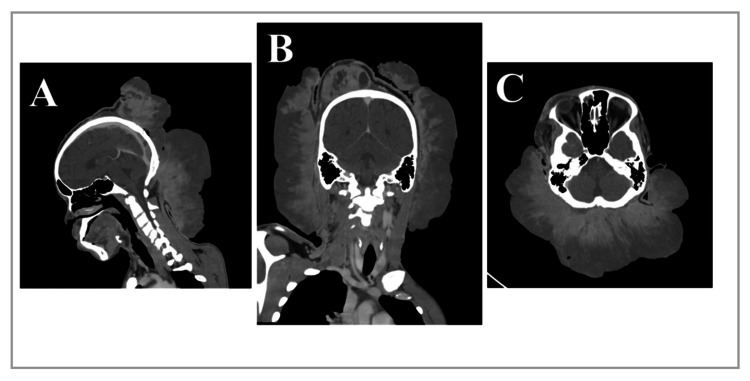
Cranial CT scan images showing a large and exophytic left parieto-occipital mass: sagittal (A), coronal (B), and axial (C) reconstructions CT: Computed tomography.
